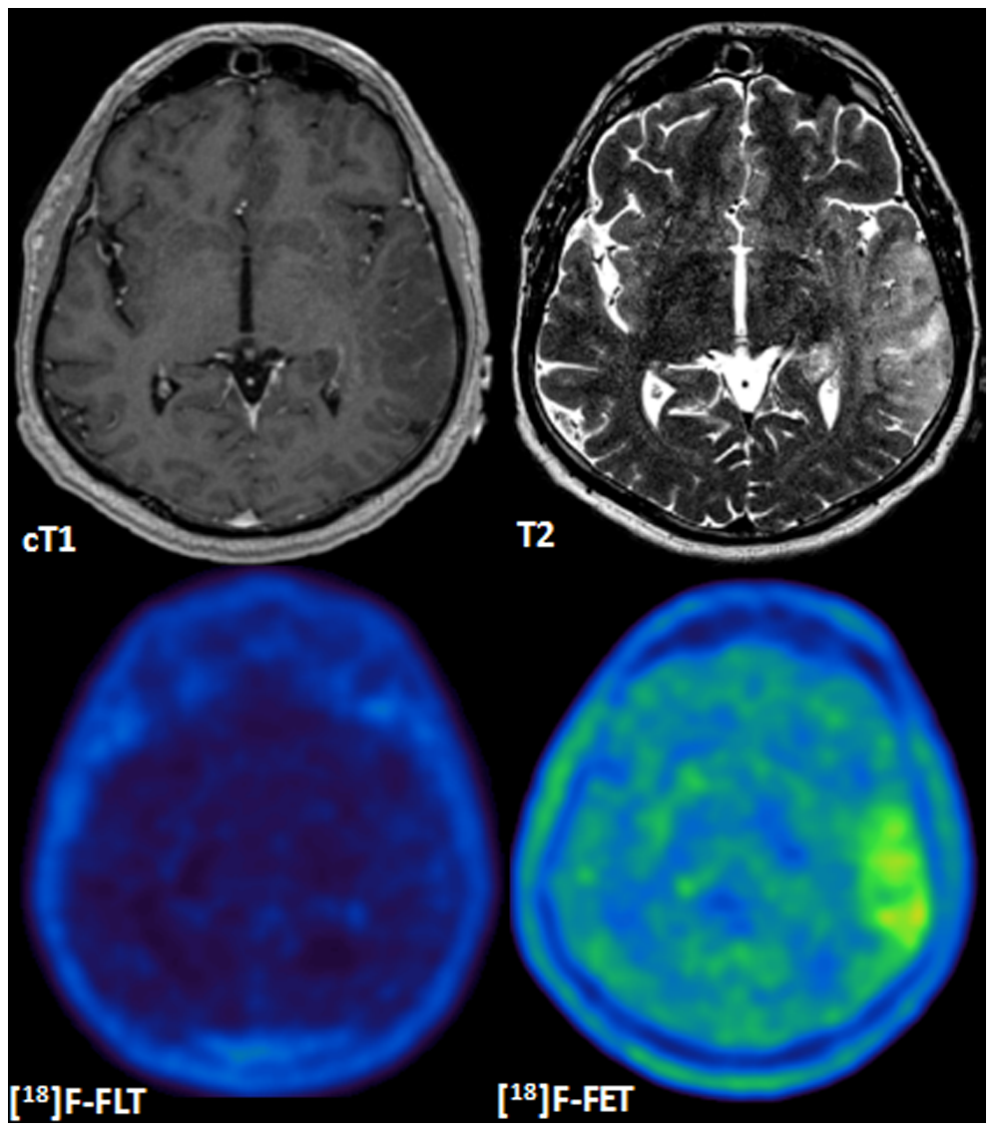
MRI and PET in a patient with non-enhancing GBM WHO IV. Newly diagnosed GBM WHO IV presenting with a hyperintense T2 lesion left parieto-temporal but without contrast enhancement on cT1. In contrast to the absence of [^18^F]-FLT tracer uptake, a metabolically active lesion can clearly be depicted on [^18^F]-FET PET.
